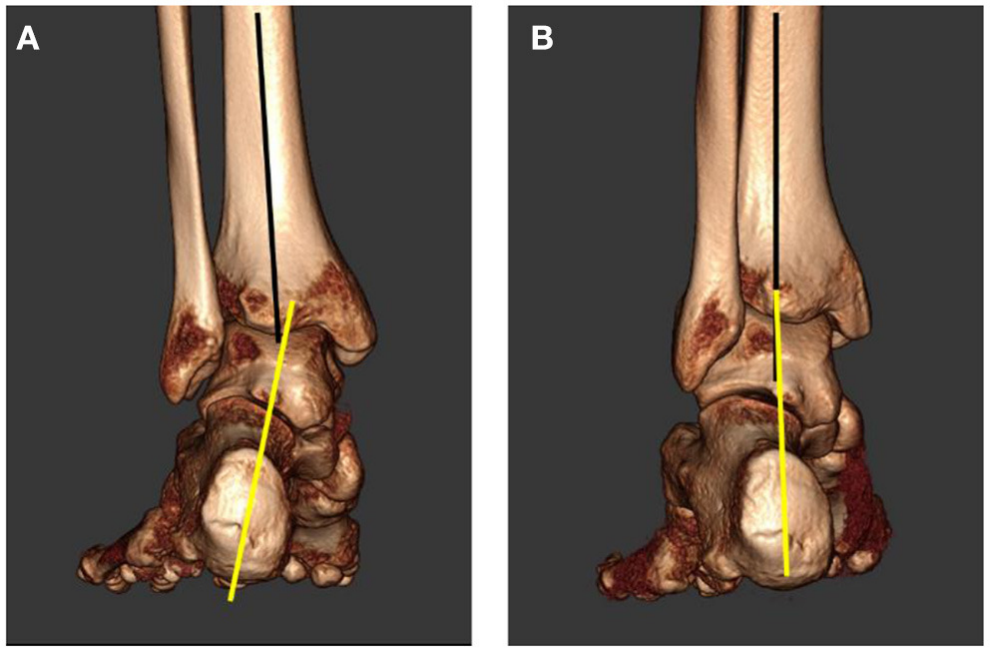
Posterior 3-dimensional weightbearing computed tomography of the hindfoot. (A) Hindfoot is in relaxed-stance position, notice the heel valgus (yellow line). (B) Same foot with the subtalar joint placed into neutral alignment, notice the rectus heel alignment.
